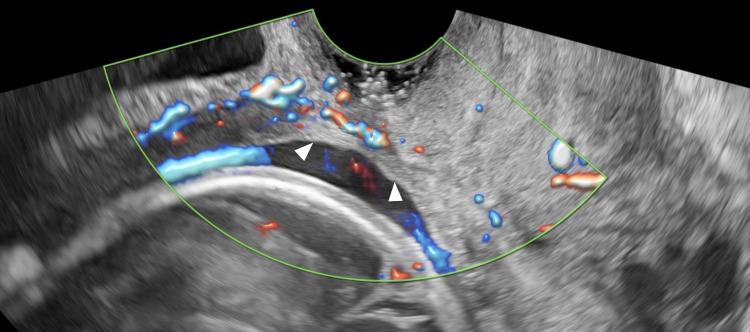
Sagittal transvaginal ultrasound color Doppler imaging at 24 weeks gestation The boundary between the decidual and chorionic tissue becomes thin and indistinct, and the area of myometrial defect is observed to widen (arrowhead).
